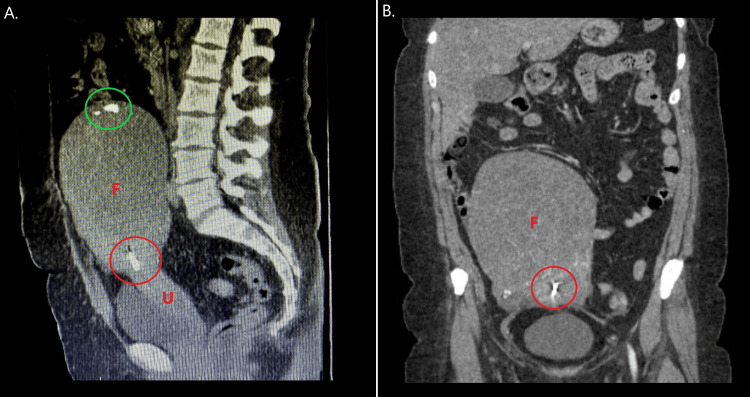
Multi-planar Computed Tomography of the patient one year prior to her presentation. A: Saggital plane, B: Coronal plane F: Fibroid, U: Uterus, red circle: intra-uterine copper device causing visual artifacts, green circle: calcifications
